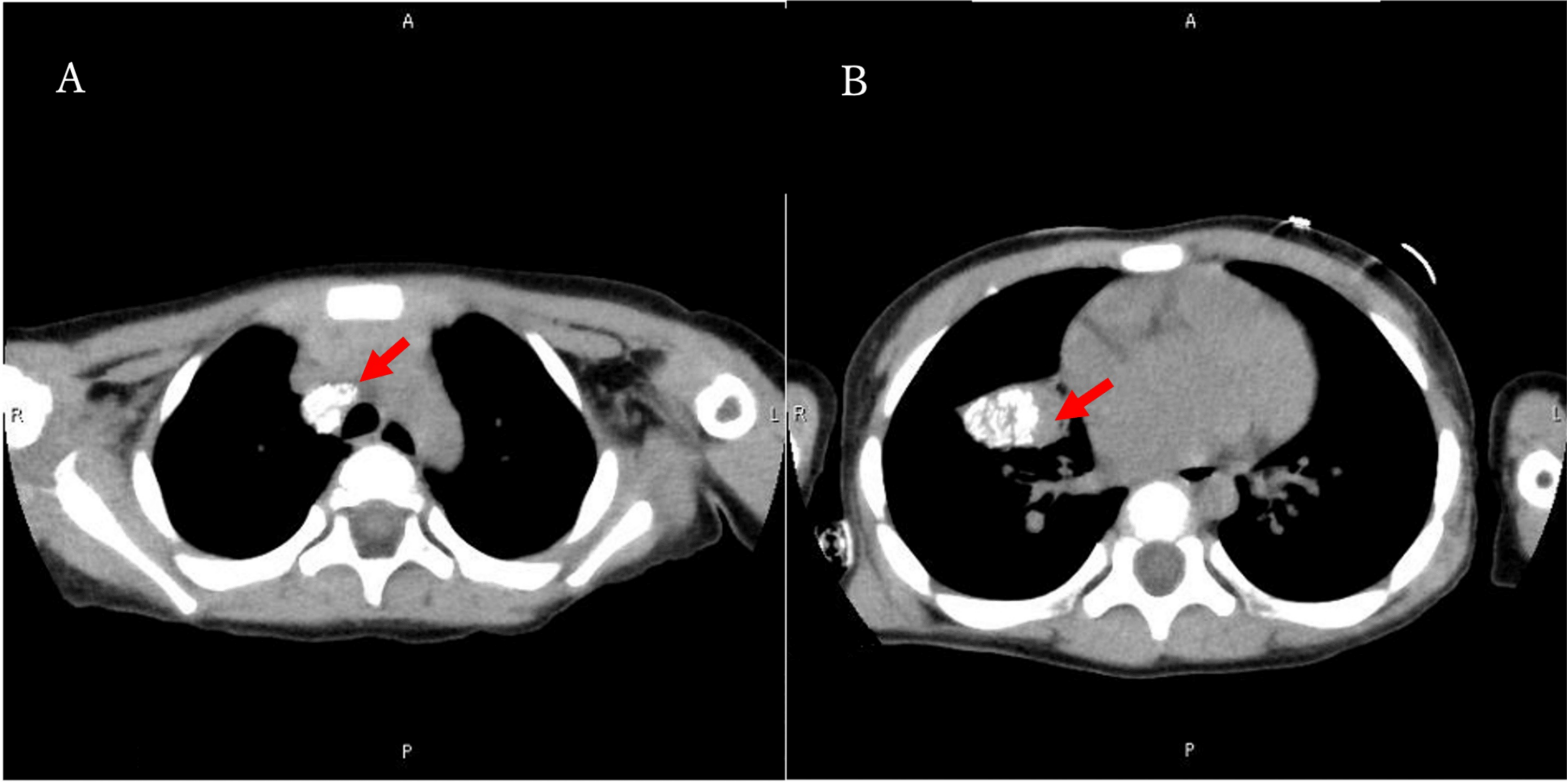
Chest computed tomography images obtained at admission. (A) Calcified lymph nodes were observed in the right pulmonary hilar region (arrow). (B) A calcified mass was detected in the right lung (arrow)
